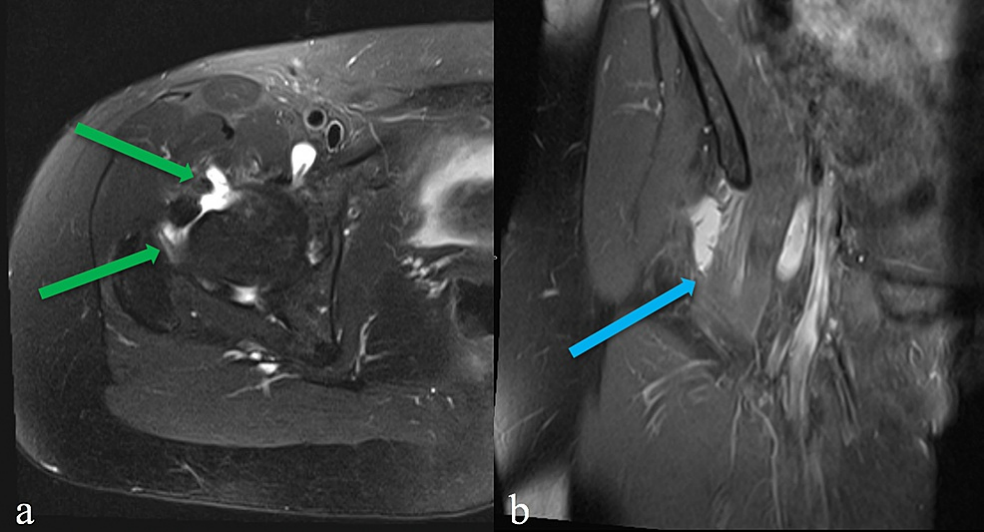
MRI of the right hip. (a) T2-axial fat-suppressed. Filling defects in the joint recesses and the iliopsoas bursa suspicious for synovial proliferation and small intra-articular bodies (green arrows). (b) Coronal proton-density (PD). Edema-like signal in the right iliopsoas muscle (blue arrow) compatible with tendinosis/tendinopathy and fluid in the iliopsoas bursa.
